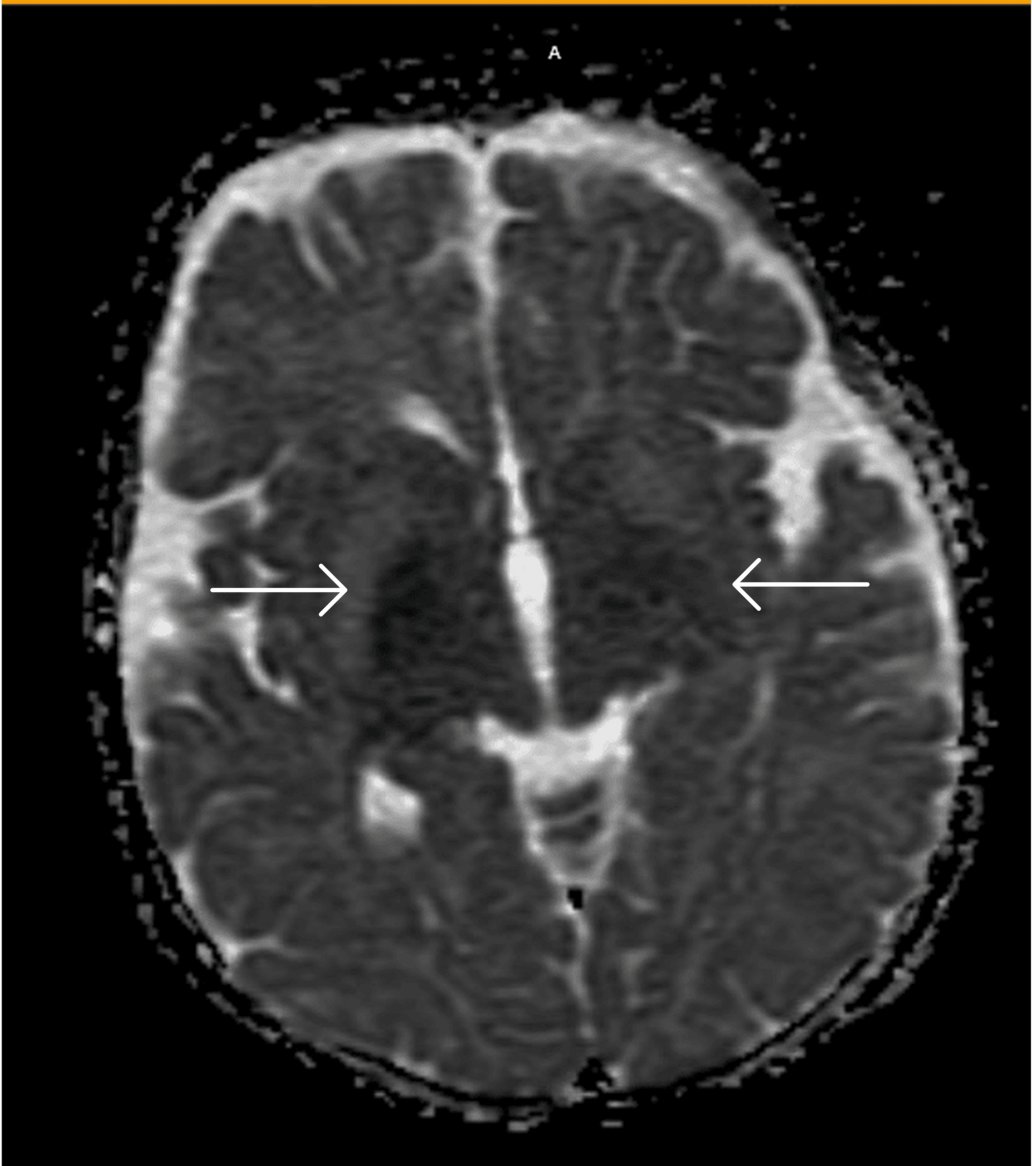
Apparent diffusion coefficient MRI showing bilateral symmetric areas of diffusion restriction mainly involving the basal ganglia including the caudate nucleus putamen and globus pallidus pallidum.
